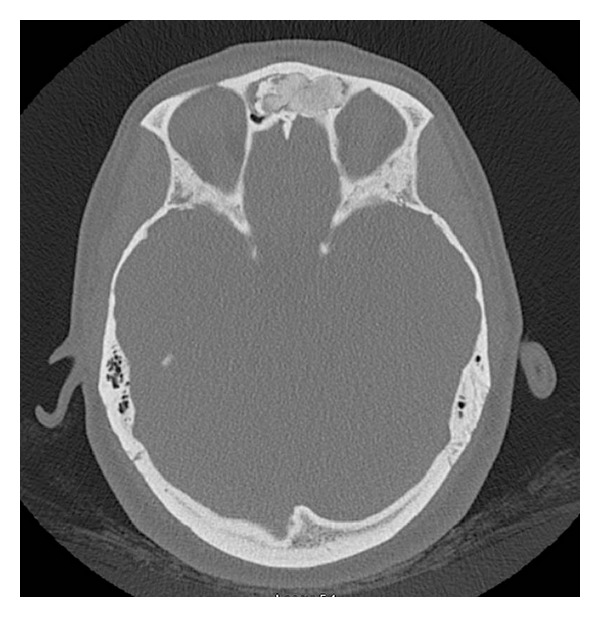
Axial CT scan showing the osseous tumor filling the frontal recess bilaterally.
